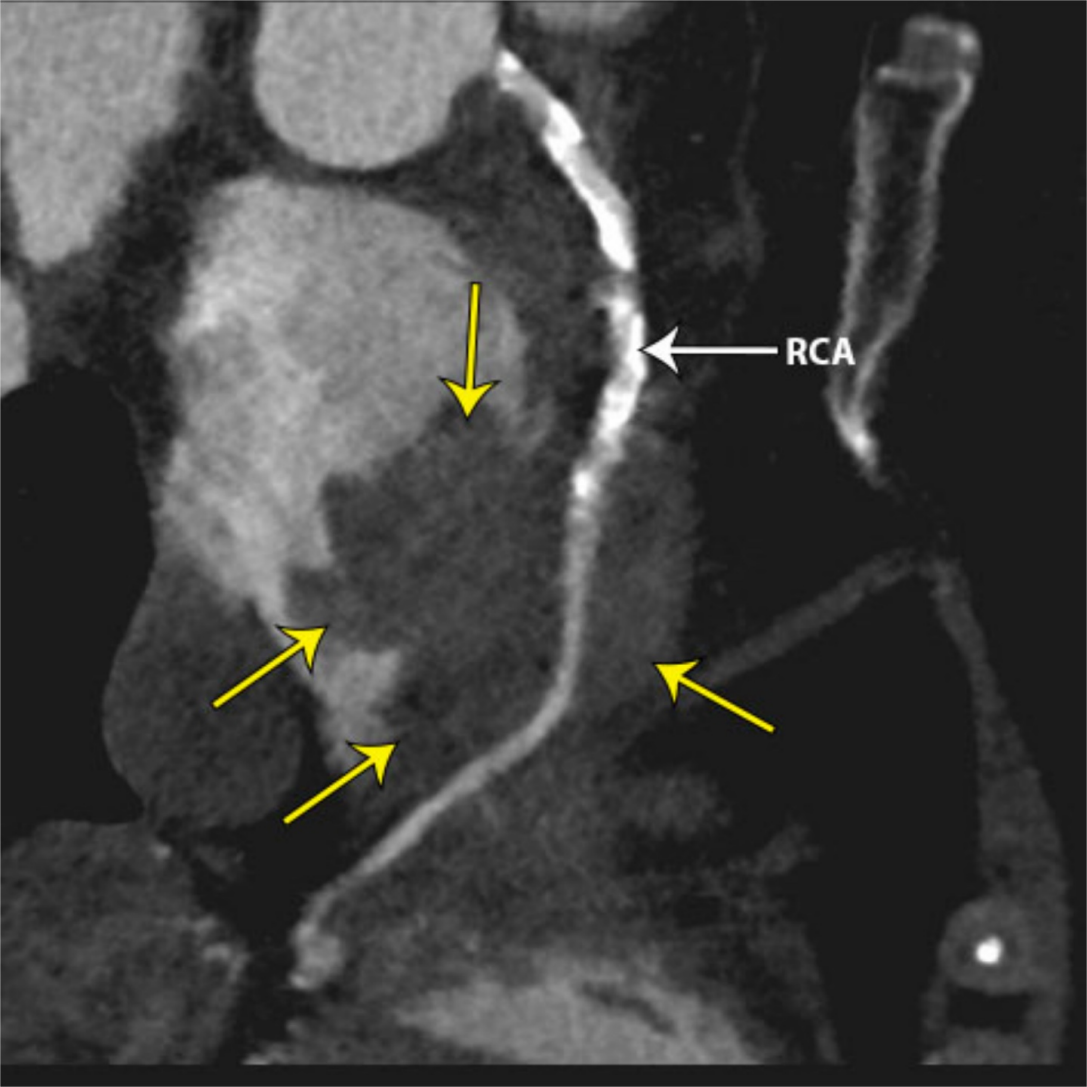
Curviplanar reformatted image of a coronary CT angiogram demonstrating a large, enhancing mass (yellow arrows) encasing most of the right coronary artery (RCA).
